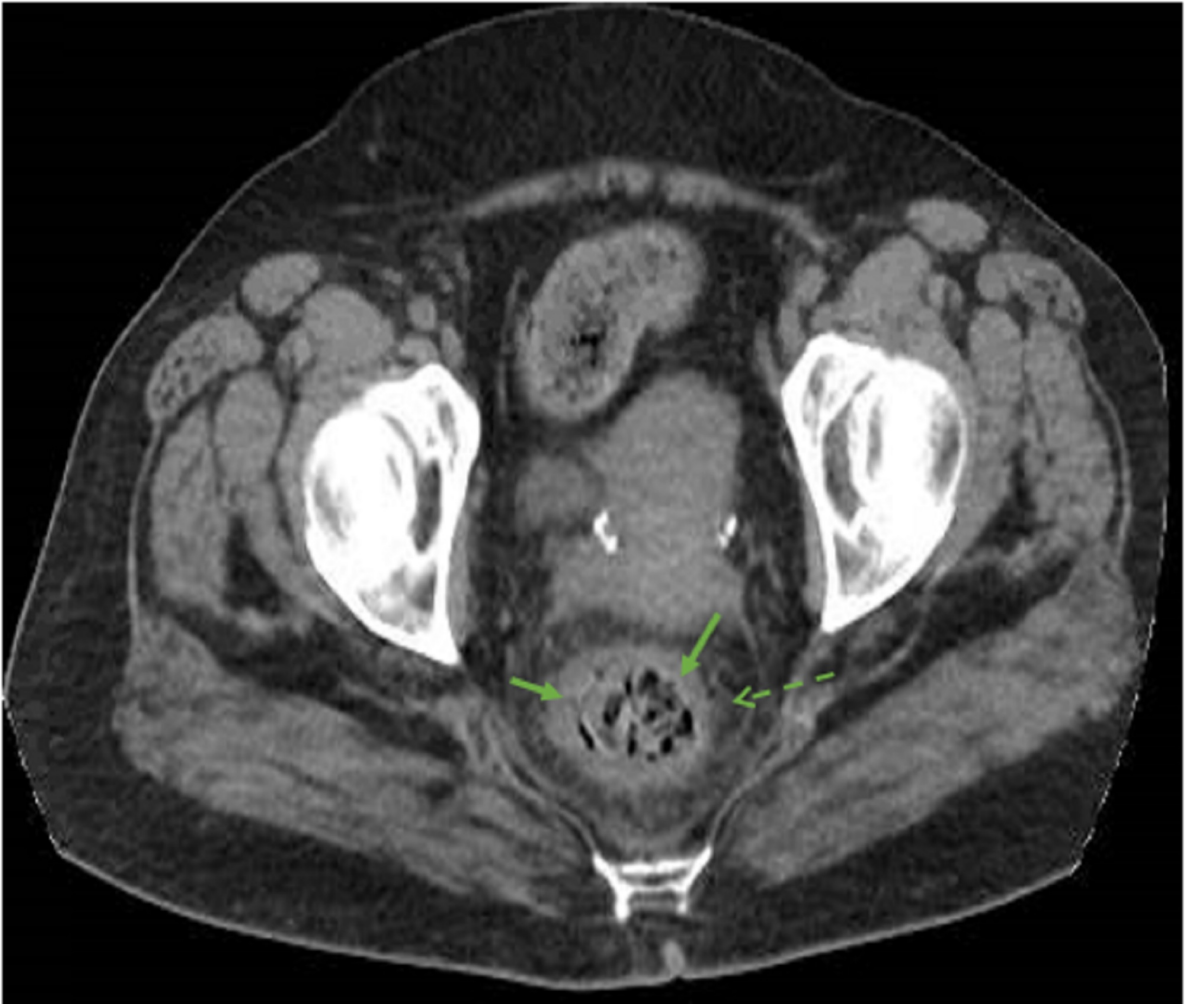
Inflammatory changes of the rectum compatible with proctitis. CT scan of the abdomen with intravenous contrast at the level of the rectum showing a circumferential wall thickening (full arrows) with fat stranding (dashed arrows) consistent with proctitis.
